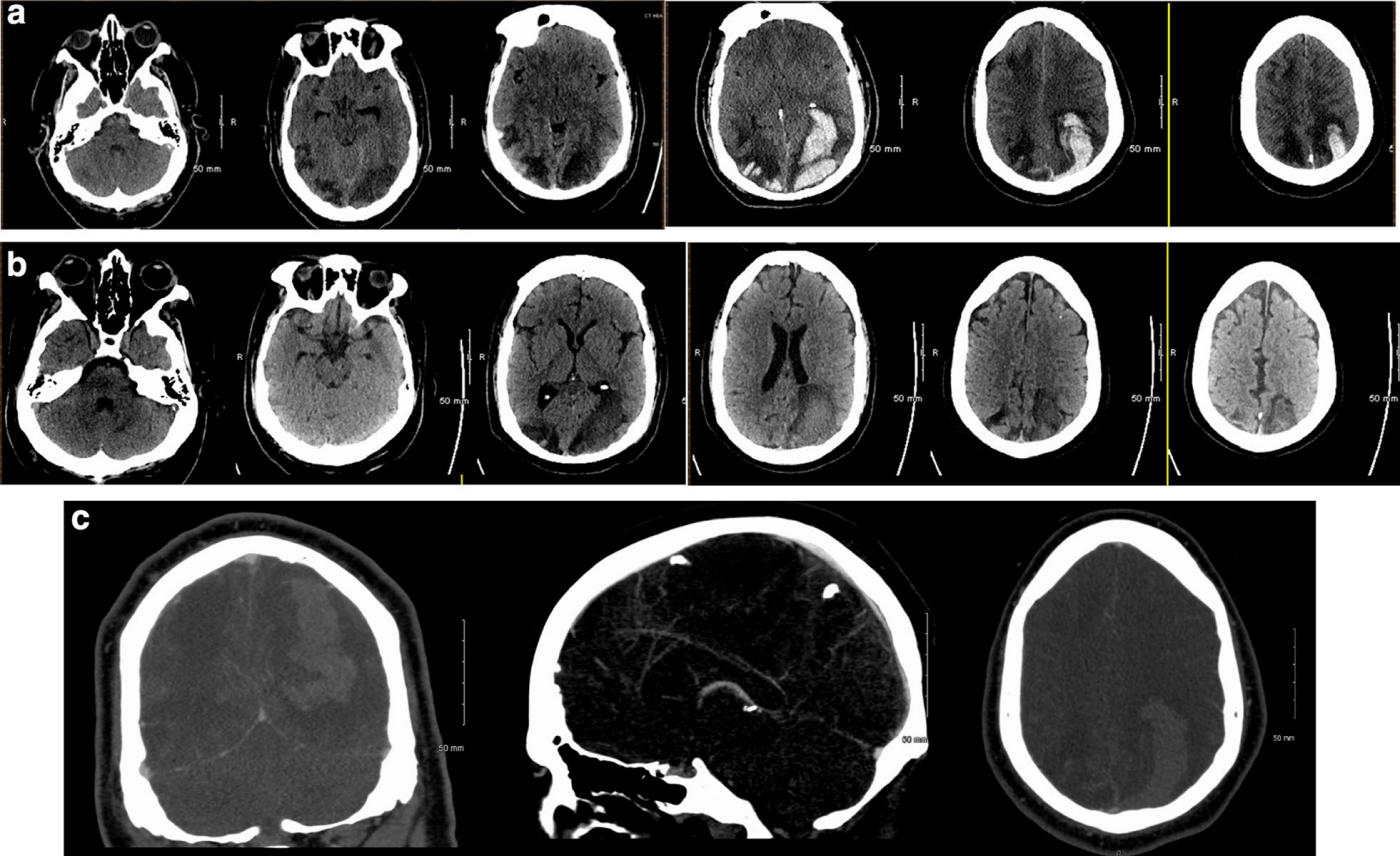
a Non-contrast CT head *(left to right: caudal to rostral)* demonstrating multifocal (particularly in the posterior circulation) cerebral edema with associated intracerebral hemorrhage, mostly centered on left > right occipital lobes and extending into the fronto-temporal region. b Non-contrast CT follow-up at 12 weeks in same sequence. c CT venography (*left*: coronal, *middle*: sagittal, *right*: axial) demonstrating patency of visualized venous system; and without any evidence of venous obstructions
